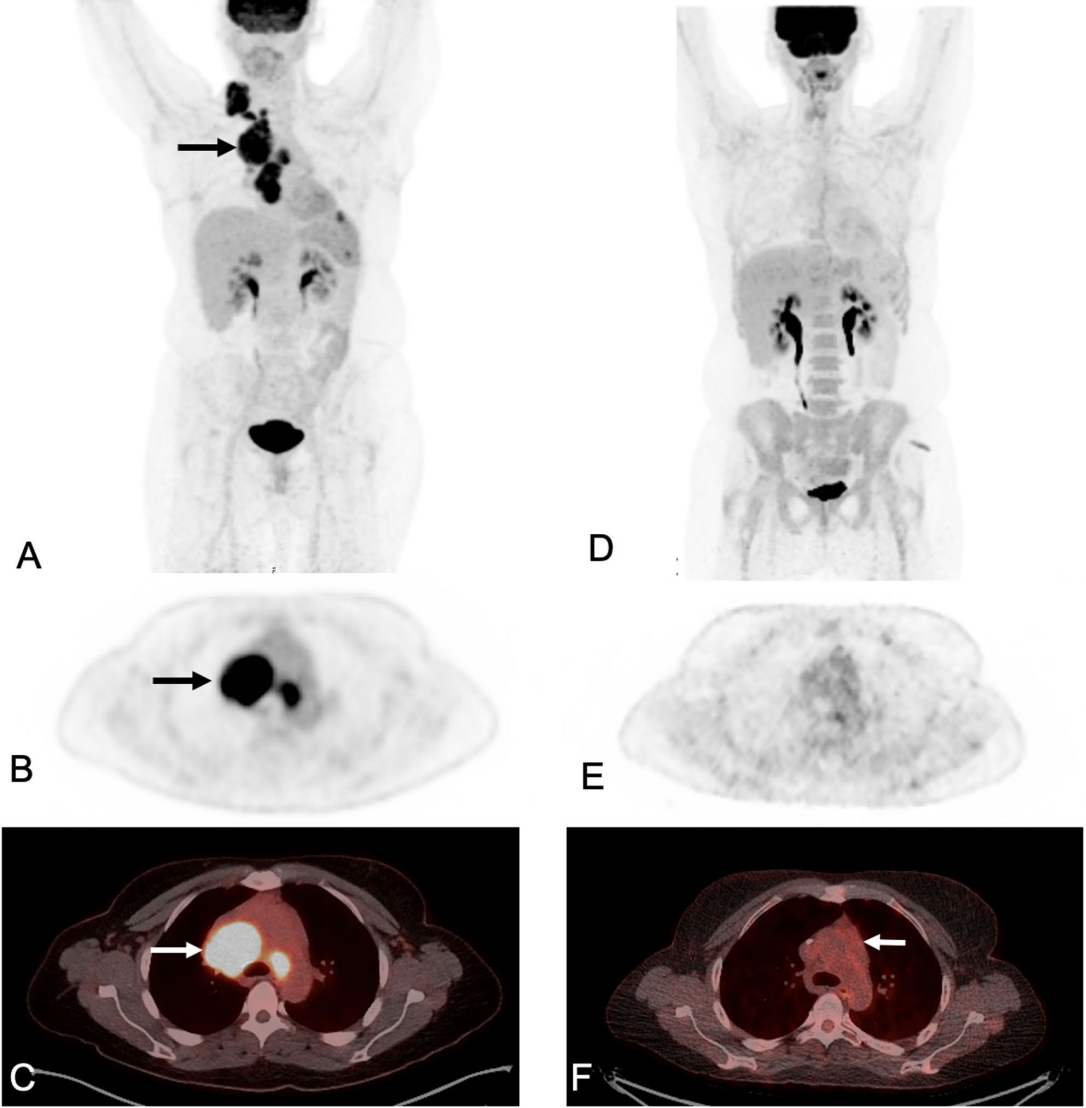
18F-FDG PET/CT of a 34-year-old female with diffuse large B-cell lymphoma who achieved a complete metabolic response after CD19/20 CAR T-cell therapy. (A–C) Baseline 18F-FDG PET/CT: A) Baseline MIP demonstrates FDG-avid thoracic lymphadenopathy (black arrow) consistent with active lymphoma. B) Axial PET demonstrates hypermetabolic anterior mediastinal lymph nodes activity (black arrow) C) Baseline fused axial 18F-FDG PET/CT confirms multiple hypermetabolic anterior mediastinal lymph nodes (white arrows). (D–F) Follow up 18F-FDG PET/CT at day 28 after CAR T-cell infusion D) Follow-up MIP at day 28 after CAR T-cell infusion shows complete metabolic response. E) Axial PET confirms complete metabolic response to therapy with decrease in size and FDG uptake of the previously noted thoracic lymphadenopathy. No new sites of FDG avid disease noted. F) Follow up fused axial 18F-FDG PET/CT confirms decrease in size and FDG uptake of multiple mediastinal lymph nodes (white arrows)
